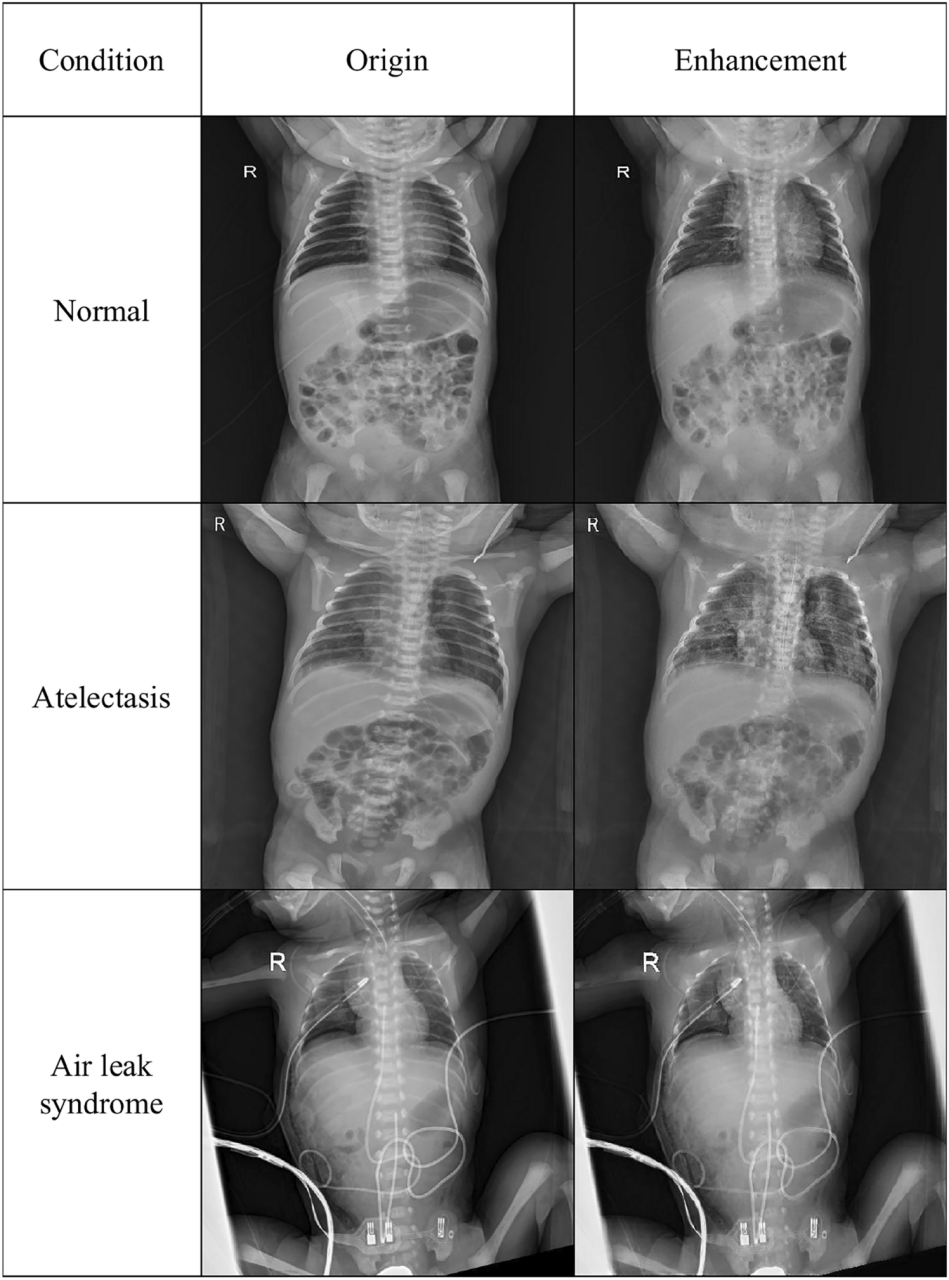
Comparison of X-ray images for different conditions with and without enhancement.
